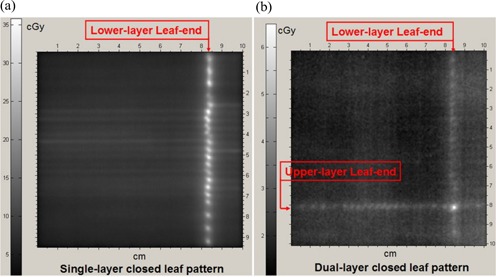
Radiographs for single‐layer and dual‐layer multileaf collimator (MLC) leaf‐end dose leakage with 6‐MV X‐rays. (a) Single‐layer closed pattern with a maximum leaf‐end transmission of 35.5 cGy. (b) Dual‐layer closed pattern with a maximum leaf‐end transmission of 6.5 cGy.
